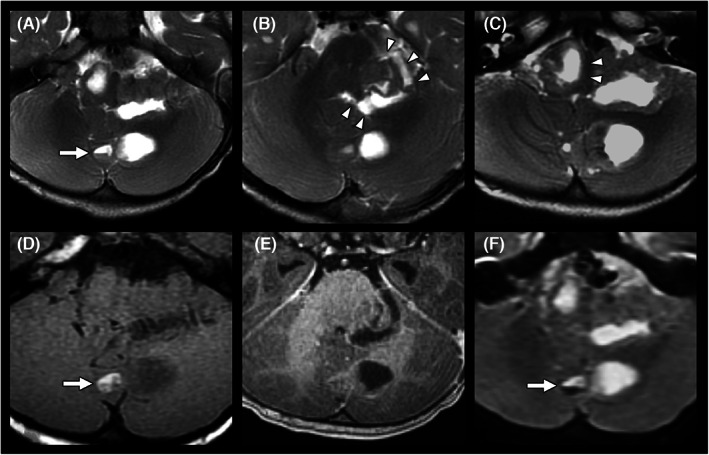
A case of atypical teratoid rhabdoid tumor (AT/RT). A 1‐year boy presented with unsteady gait for 2 weeks. On presurgical MRI, T2WI revealed a multiloculated mass lesion with central necrosis in the posterior fossa (A–C). The lesion showed local invasion into the middle cerebellar peduncle (B, arrowheads) and brain stem (C, arrowheads). The soft tissue components appeared isointense on T1WI (D) and showed good enhancement on contrast‐enhanced T1WI (E). A fluid–fluid level indicating intralesional hemorrhage was observed on T2WI (A, arrow), T1WI (D, arrow), and DWI, with *b* = 0 s/mm^2^ (F, arrow). The pathological report disclosed atypical teratoid rhabdoid tumor with focal tumor necrosis.
